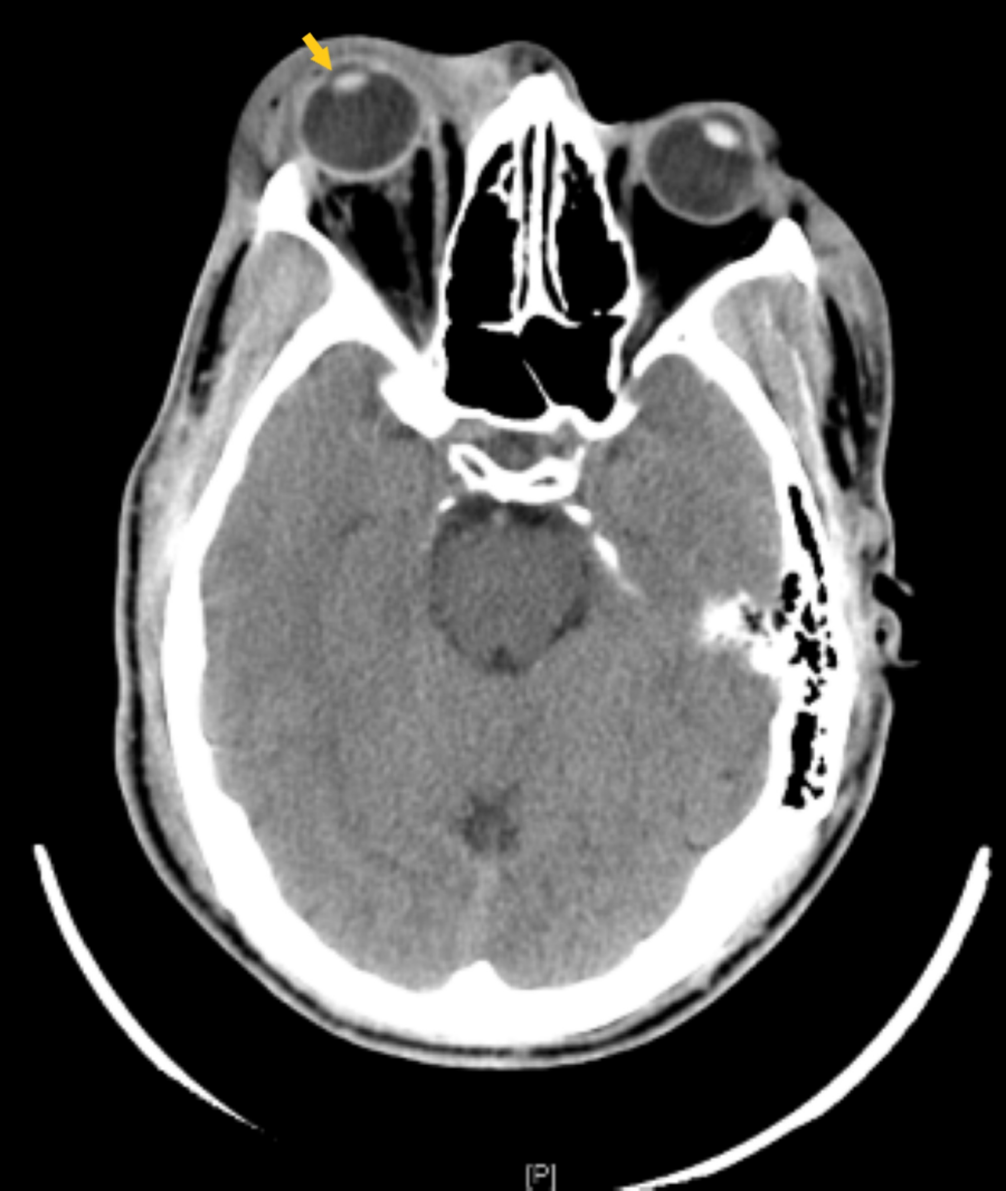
CT demonstrating right exophthalmos (arrow) with intraorbital fat stranding and abnormal caliber and density of the optic nerve sheath complex. Also seen is right preseptal subcutaneous soft tissue swelling and hematoma.
